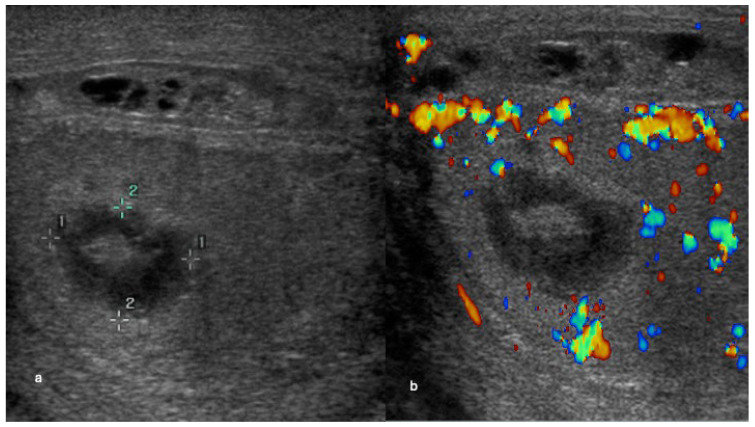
Avascular malignant testicular lesion (acute myeloid leukaemia involvement). Longitudinal images of the left testis: (a) grey-scale ultrasound shows a small focal intratesticular lesion (15 × 10 × 10 mm; callipers); (b) colour Doppler ultrasound demonstrates no detectable intralesional vascularity.
